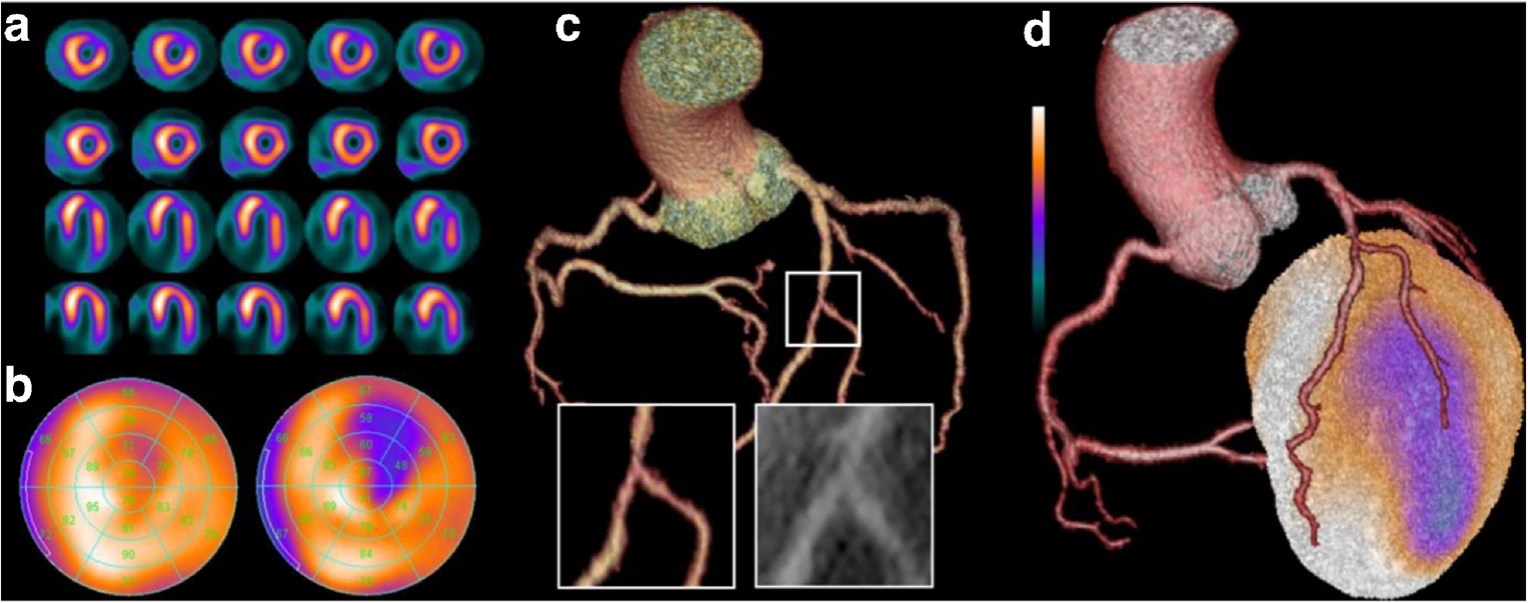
Coronary artery territories in a 17-segment model Myocardial perfusion SPECT, coronary computed tomography angiography (CCTA), and fused hybrid SPECT/CCTA of a 43-year-old male patient with presenting symptoms of typical angina. Myocardial perfusion SPECT documents a reversible perfusion defect in short axis and horizontal long axis slices (a) at rest (*bottom rows*) and stress (*top rows*). The corresponding polar plots (b) at rest (*left plot*) and stress (right plot) clearly depict the extent of the ischaemic area in the anterolateral wall. CCTA (c) shows an intermediate stenosis (i.e., 50–70 % luminal narrowing) due to non-calcified plaques in the middle/distal left anterior descending artery at the level of the second diagonal branch bifurcation. Fused hybrid SPECT/CCTA (d) reveals that the anterolateral ischaemia corresponds with the vascular territory of the second diagonal branch, while the stenosis in the left anterior descending artery (LAD) does not cause any ischaemia
